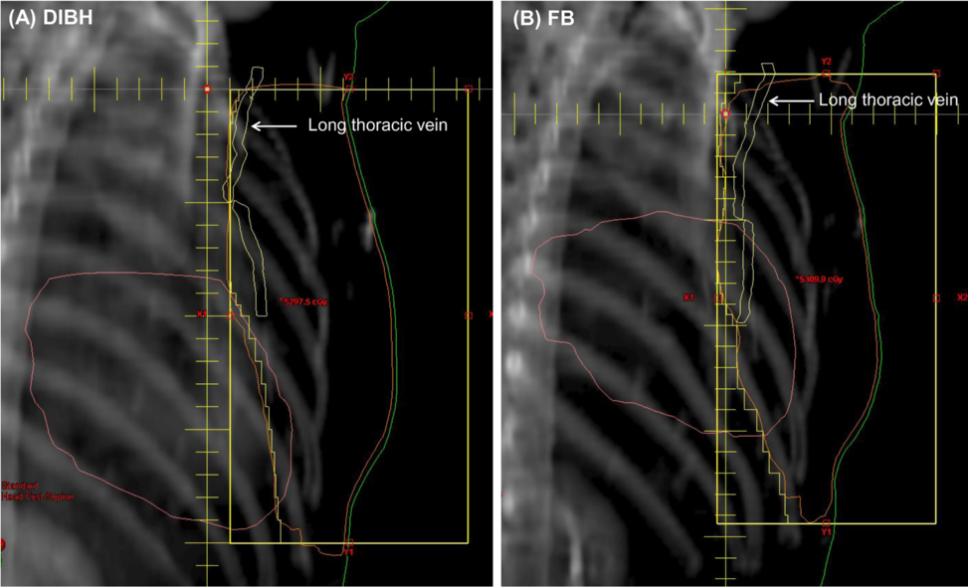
Digitally reconstructed radiographs of the deep inspiration breath holding (DIBH) (a) and free breathing (FB) (b) techniques. Posterior–inferior displacement of the cardiac apex and decreased maximal heart distance are shown during DIBH
